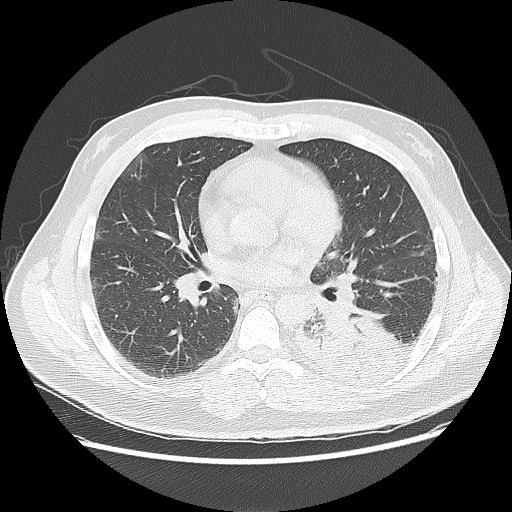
Chest CT demonstrating left-sided pneumonia.
